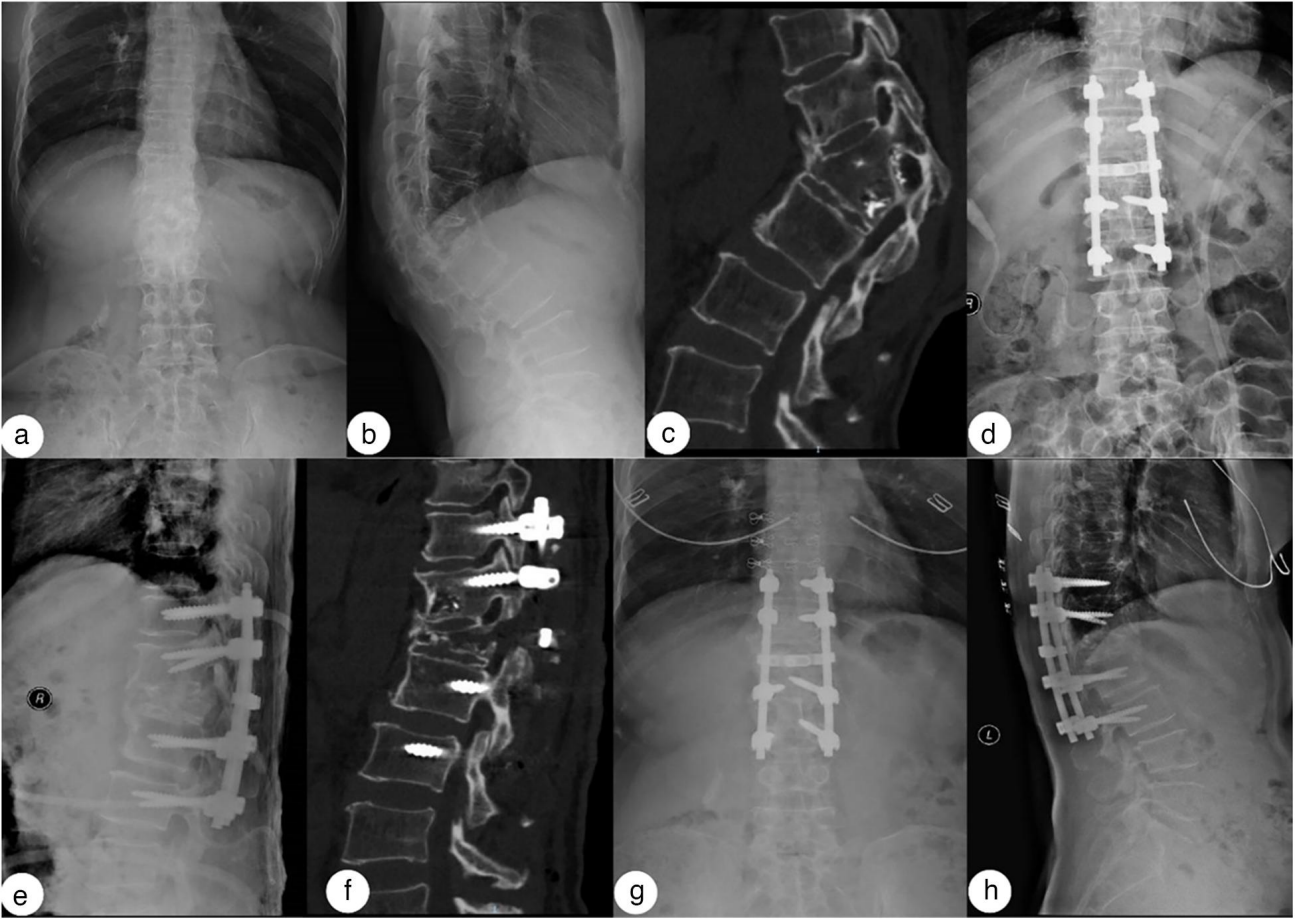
A 52-year-old woman who developed kyphosis after a T12 fracture caused by a fall. The VAS score was 9. (a,b) A preoperative X-ray shows a long-standing compression fracture of the T12 vertebral body, with a kyphosis Cobb angle of 54.85°. (c,d) Intraoperative operation and fluoroscopy. (e,f) Postoperative correction was satisfactory, with a kyphosis Cobb angle of 19.98°. (g,h) At the 1-year follow-up, there were no complications such as broken nails or rods. The osteotomy surface was fused, and the kyphosis Cobb angle was 19.67°.
